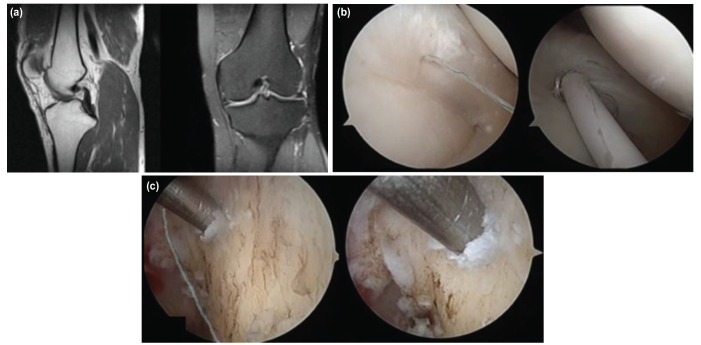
The 28-year-old male patient has ACL injury and meniscus tear in his left knee, (a) Sagittal and axial section MR image, (b) repair of the medial meniscus tear with all inside suture, (c) Placement of the guidewire, view of the distance to the inferior and posterior cortex (blue line).
